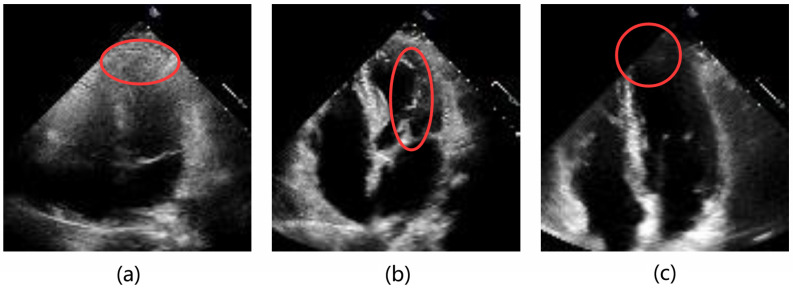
Several challenges in echocardiography image segmentation: (a) Blurred contours due to speckle noise. (b) Ambiguous contours caused by papillary muscle. (c) Contours out of the field of view.
